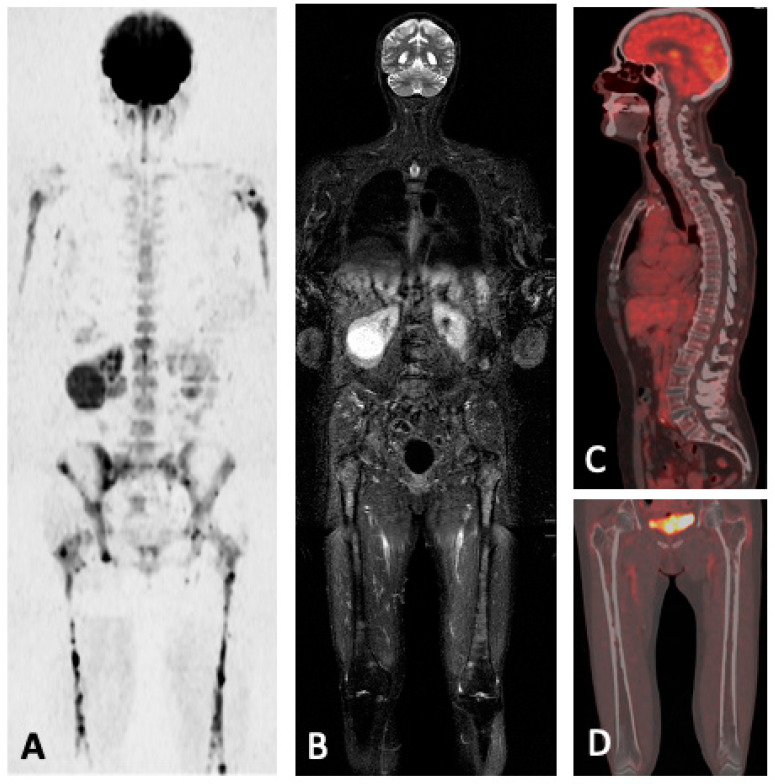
A 70-year-old woman with multifocal involvement of MM in the axial and peripheral skeleton. Multiple lesions with high SI in *b* = 900 DWI (A) and high SI in STIR (B). Follow-up FDG-PET/CT (C,D) shows successful post-treatment changes with no pathological FDG uptake.
